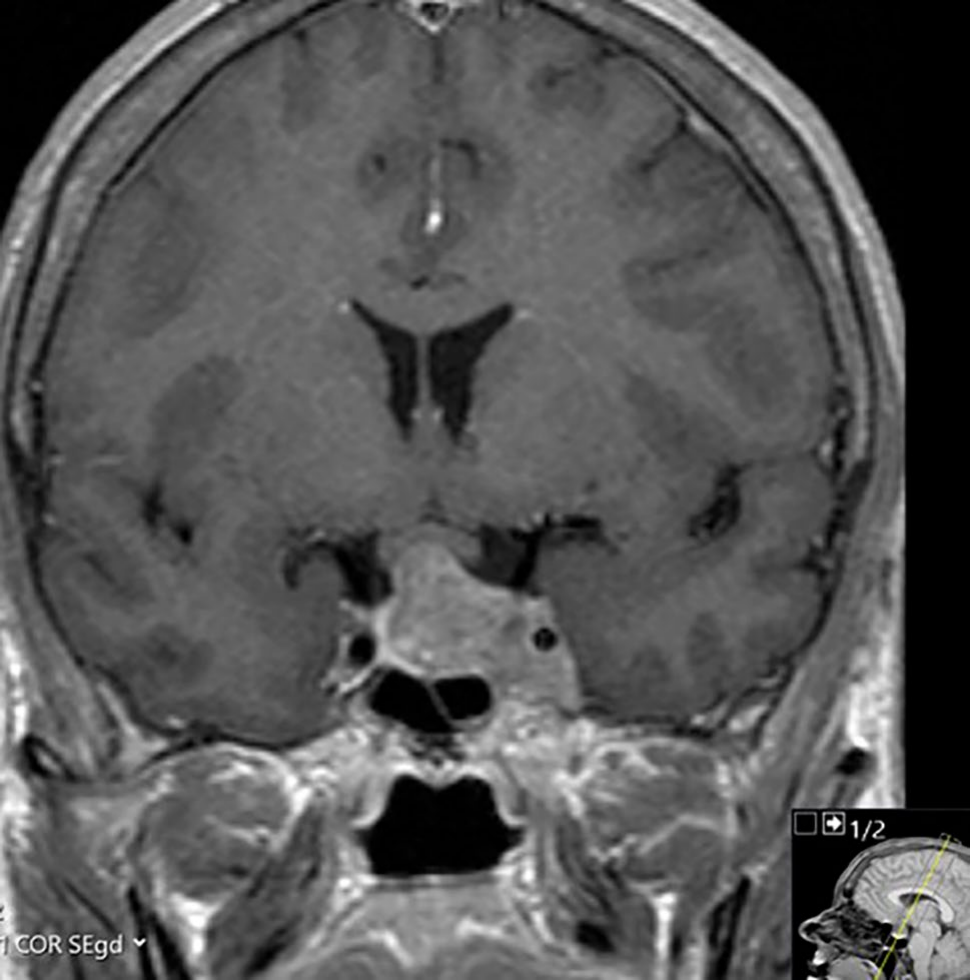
Magnetic resonance imaging of the sellar region. In 2008, the diagnostic coronal T1-weighted magnetic resonance image of the sellar region after intravenous administration of contrast revealed a macroadenoma with invasion of the left cavernous sinus and some compression of the optic chiasm
